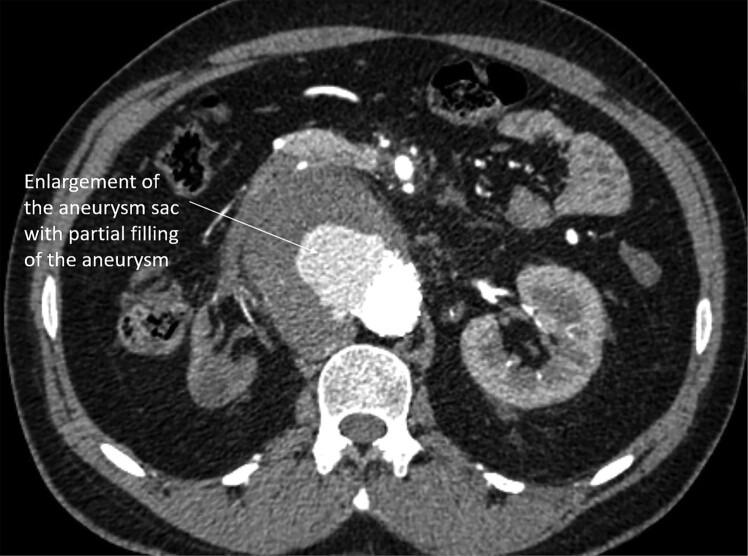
Follow-up CT. CT after two years showed contrast enhancement in the abdominal aortic saccular aneurysm and enlargement of the aneurysm.
